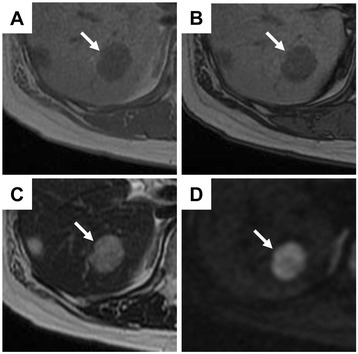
Magnetic resonance imaging (MRI). A. In-phase image of chemical shift T1-weighted MRI shows low intensity at the tumor of segment 6 (*arrow*). B. No further decrease of signal intensity was observed in out-of-phase image (*arrow*). C. T2-weighted MRI shows high intensity (*arrow*). D. Diffusion-weighted imaging showed high intensity at the tumor (*arrow*).
